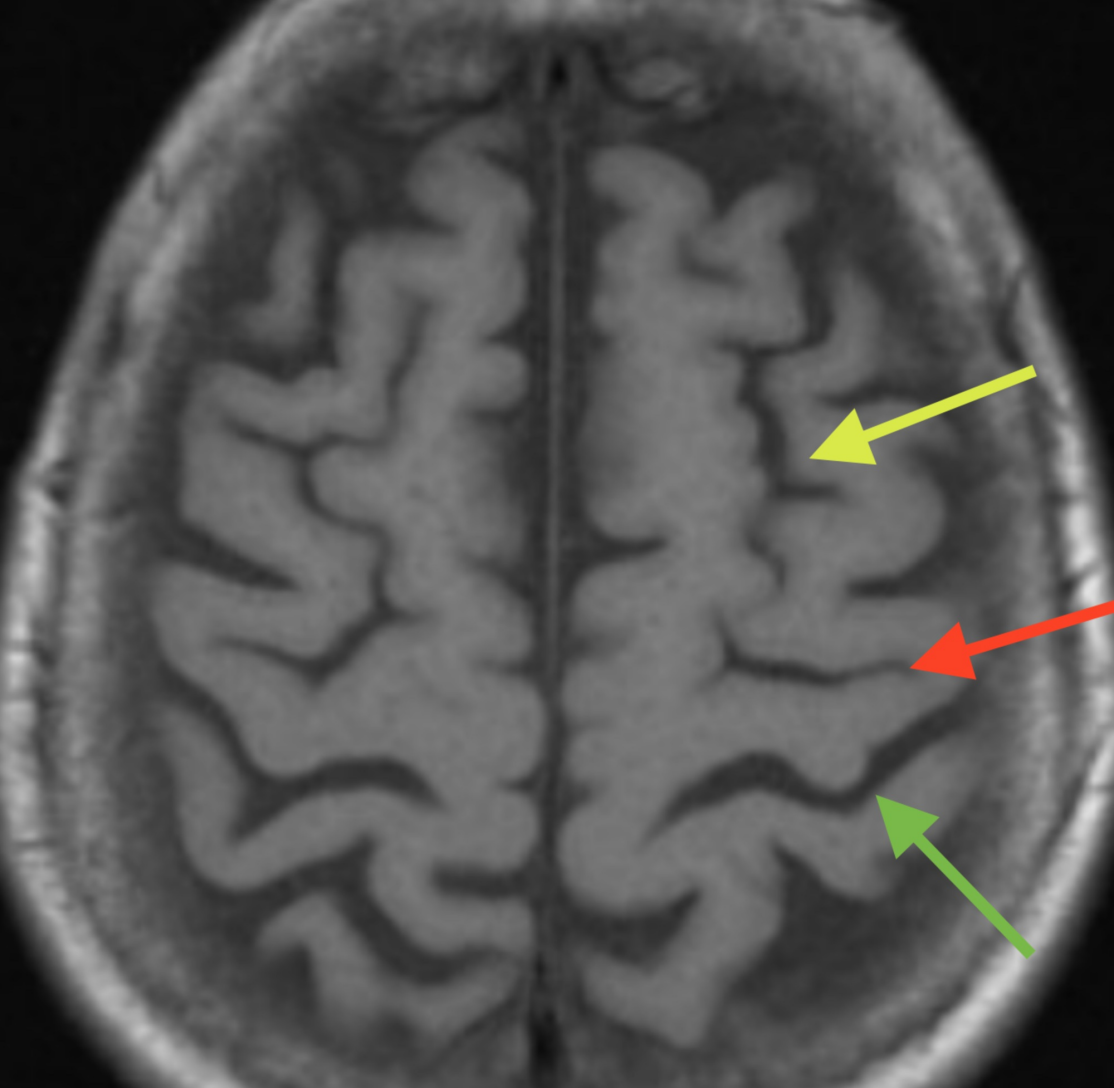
T1-weighted axial MRI The image shows superior frontal sulcus (yellow arrow), central sulcus (red arrow), and omega sulcus (green arrow) MRI: magnetic resonance imaging
